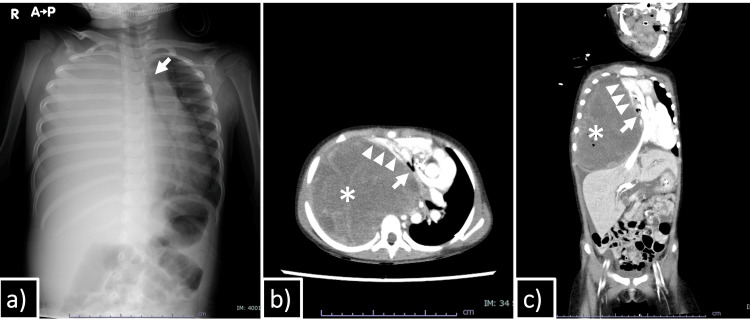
Chest X-ray and contrast-enhanced CT Chest radiograph obtained on the day of referral showed complete opacification of the right hemithorax and deviation of the trachea (arrow) (a). Axial (b) and coronal (c) contrast-enhanced CT images demonstrated a large tumor (asterisk) occupying the entire right thoracic cavity, with the normal lung parenchyma (arrowheads) displaced anteromedially. The trachea (arrow) was deviated and markedly narrowed. CT: computed tomography
